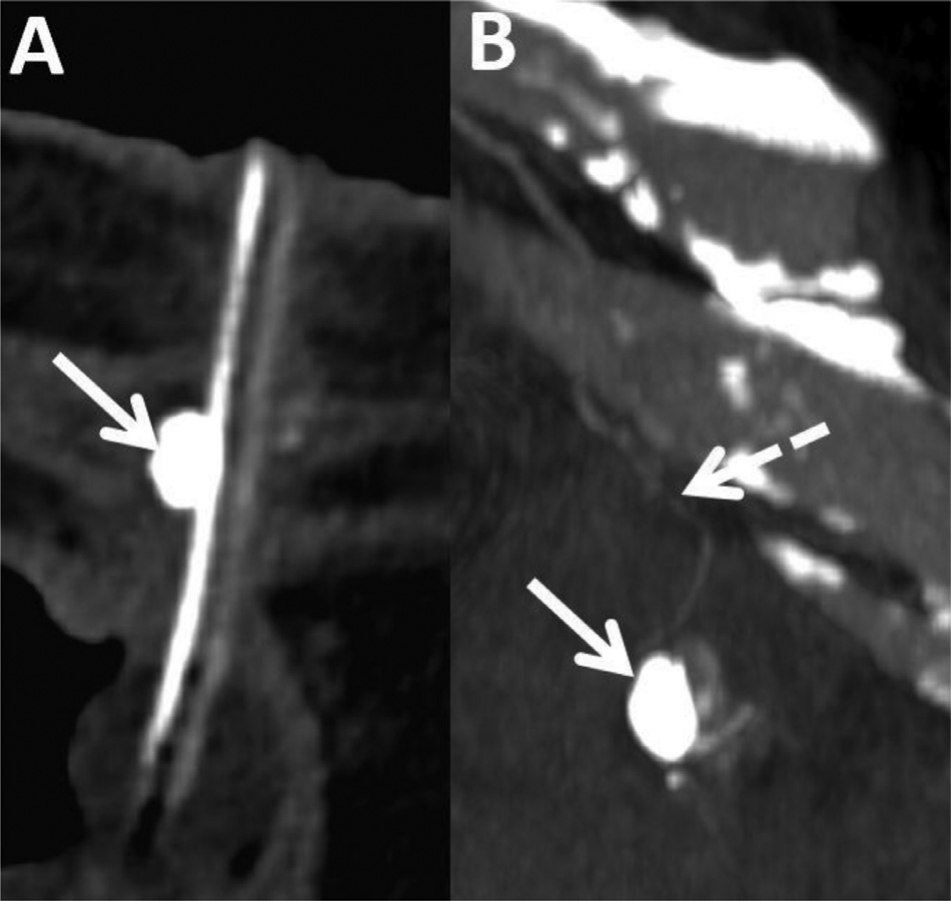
Abdominal CT scan without injection in axial section (A) confirms the complete filling of the pseudoaneurysm with the Lipiodol/Glubran2 mixture (white arrow). The coronal section scan (B) injected at arterial time shows the preserved permeability of the upper epigastric artery (dotted arrow).
